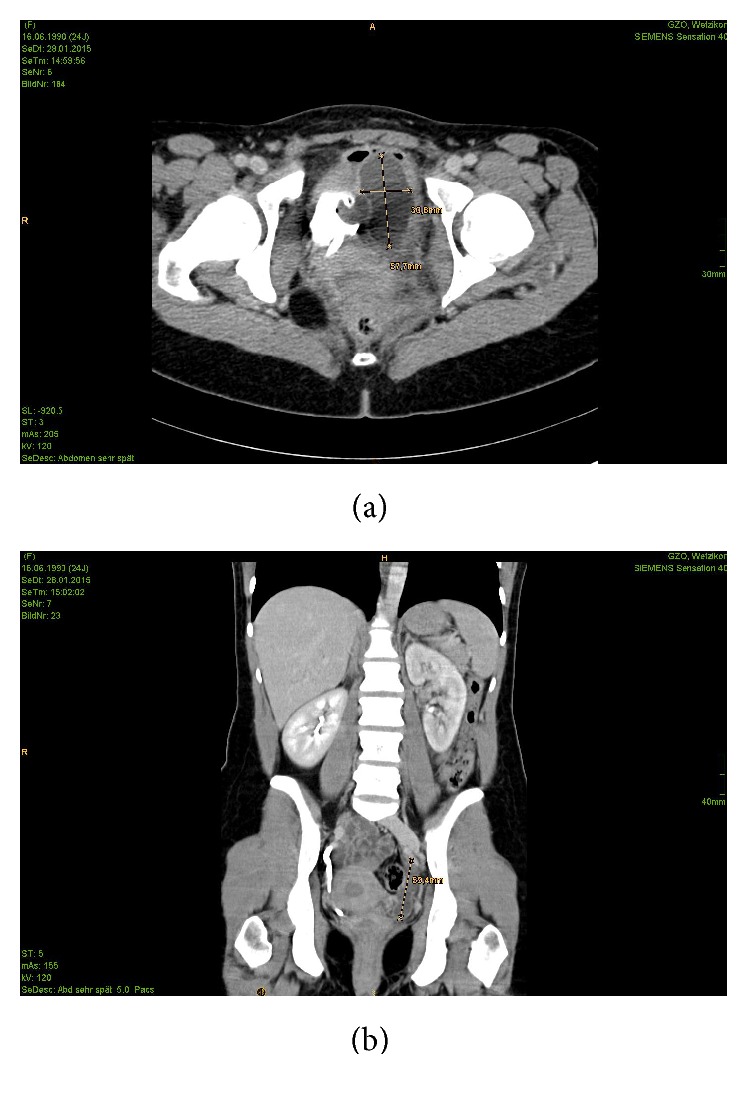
Transversal (a) and frontal (b) section of the patient's CT scan. In (a), the bladder, filled with contrast and a transurethral catheter being in place, can be found shifted to the right patient's side. The ureterocele right next to the bladder measures about 3 × 6 cm. (b) shows the dilated left ureter and that there was no incidence for a duplex renal system.
